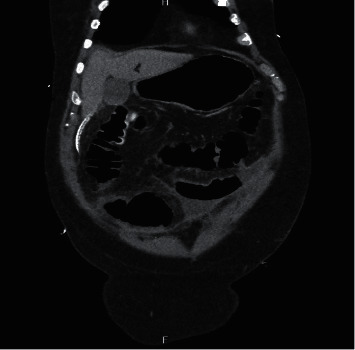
CT abdomen showing extravasation of contrast material.
